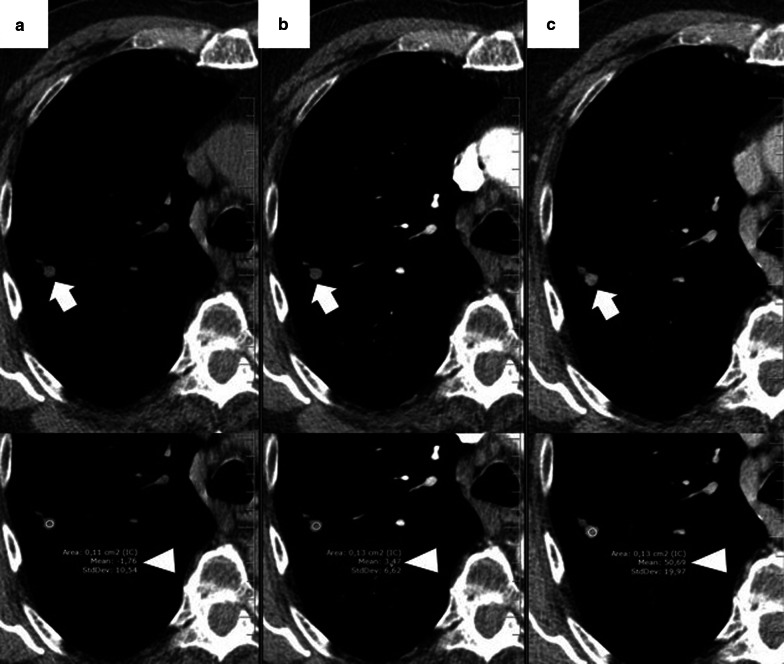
Contrast enhancement in a nodule adjacent to the treated area after image-guided percutaneous lung ablation. a Unenhanced CT showing a nodular lesion adjacent to an area treated with a lung ablation (arrow). b, c The lesion enhances after administering intravenous iodine contrast (arrows), successively increasing HU between the arterial and venous phase (triangles). These findings are compatible with tumor recurrence
